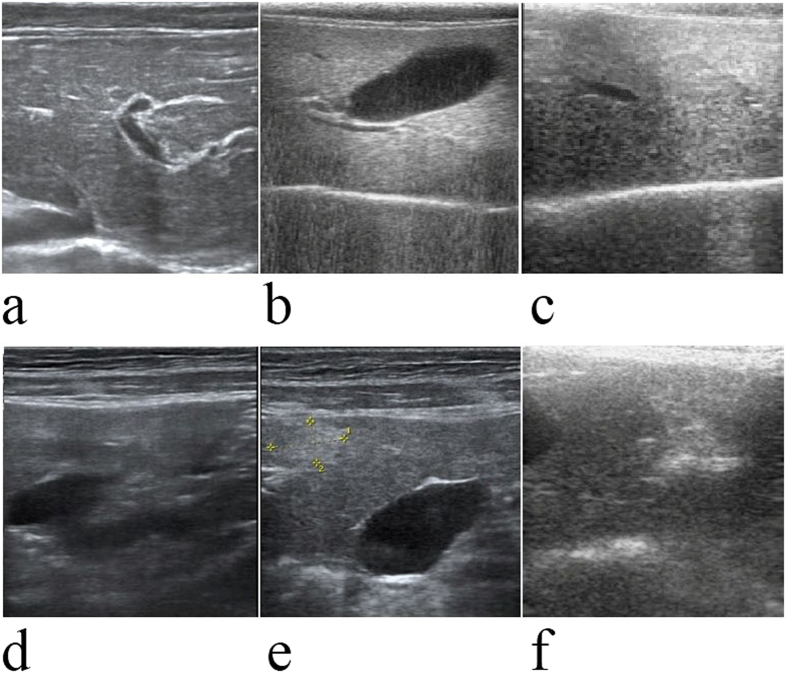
Ultrasound images of normal liver, fatty liver and liver with fibrosis. Normal liver tissue ultrasonogram (a): the liver capsule is neat and smooth with a linear, slim, strong echo, and there is a small gap in the linear echo at the peritoneal wall. The liver parenchyma spots are fine and uniformly distributed, the intrahepatic duct system is normal, the texture is clear, and entrant sound is good. Fatty liver tissue ultrasonogram (b,c): the liver capsule is neat and smooth, and the liver edge is obtuse. Intrahepatic echoes are fine and closely woven with uneven echoes. Intrahepatic vessels were significantly decreased. Liver fibrosis tissue ultrasonogram (d–f): the liver surface (d) is irregular and rough, and the liver parenchyma is uneven with irregular necrotic foci (e,f).
